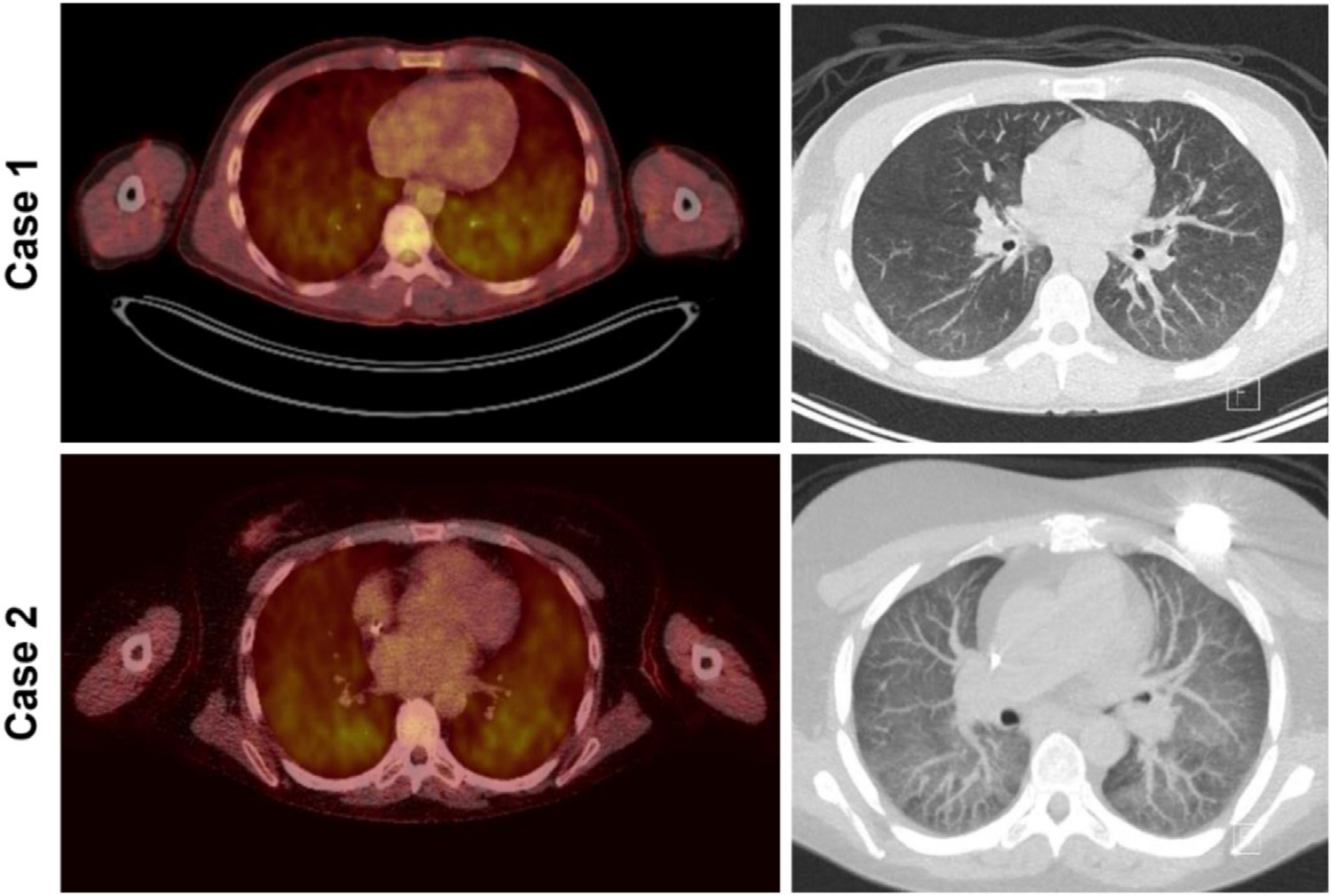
Representative PET-CT scan findings of case 1 (upper panels) and case 2 (lower panels). CT, computed tomography; PET, positron emission tomography.
